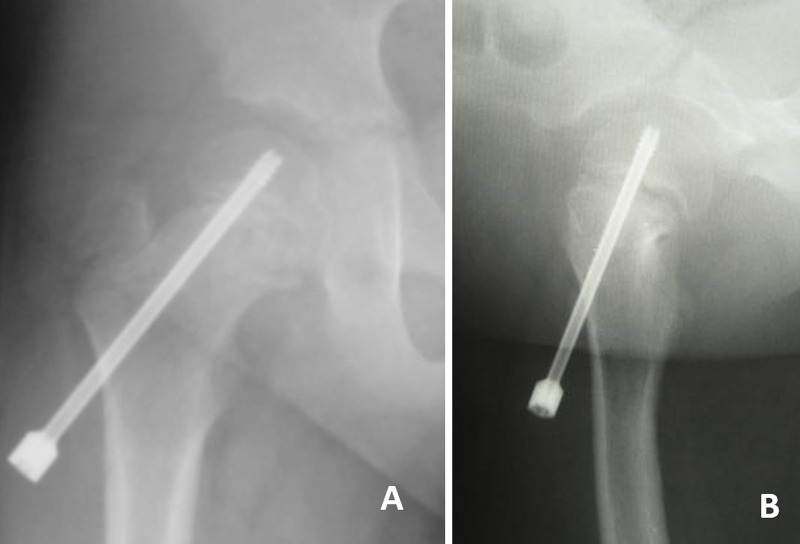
Postoperative imaging (first surgery). (A) Anteroposterior and (B) Rauenstein radiographs of the right hip following in situ dynamic single screw fixation.
